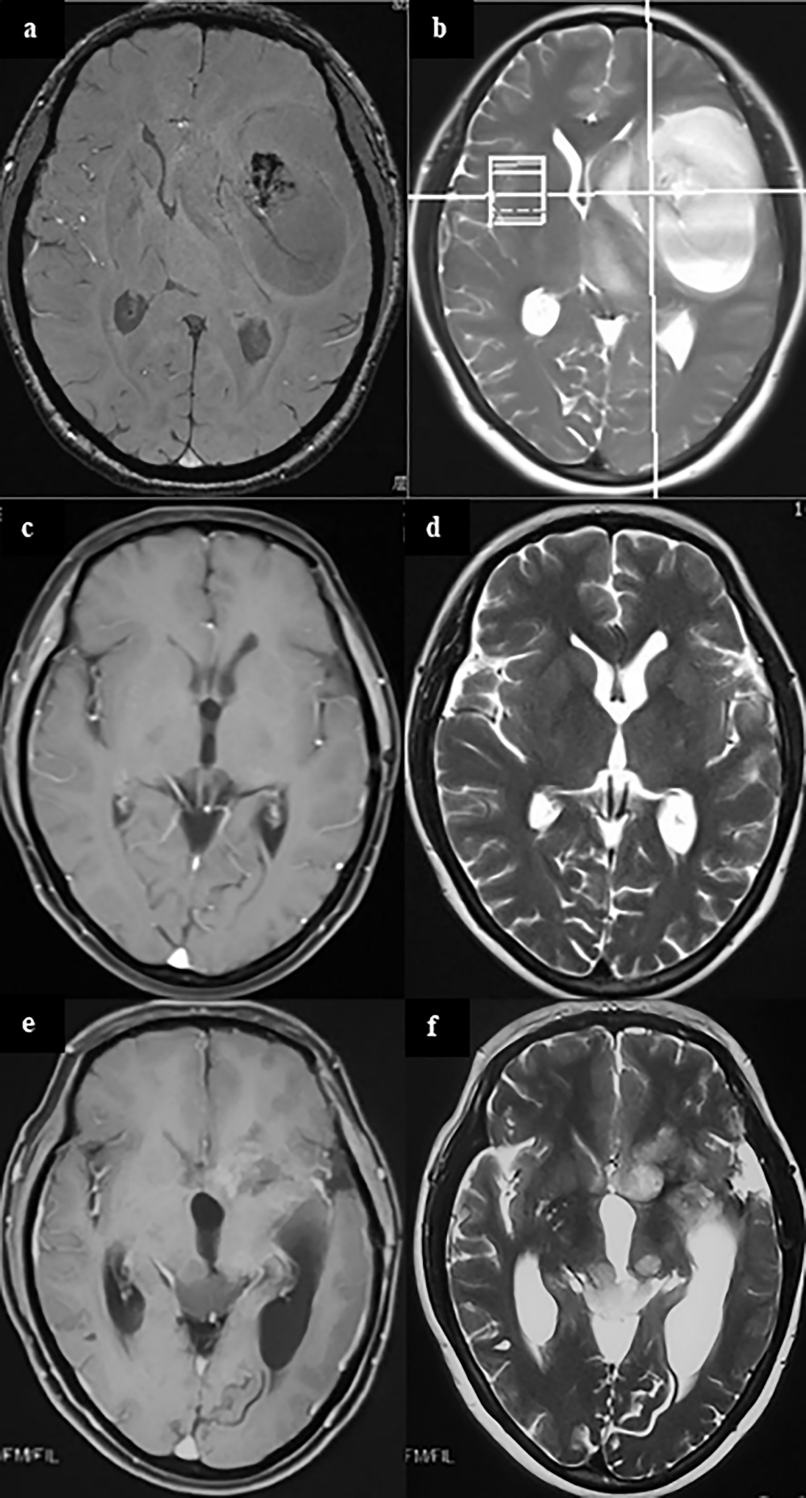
A case of metachronous multicentric glioma. The patient underwent MRI for headache and found a tumor in the left temporal insula (A, B). She was given partial resection of the tumor. Pathology showed GBM. Local radiation and temozolomide based chemotherapy were given after surgery. The tumor completely regressed in MRI 1 year after surgery (C, D). However, in follow-up 1.5 years after surgery, it was found that the patient had a recurrent lesion in the left caudate head and insula, and an ectopic lesion in the midbrain tectum (E, F). On sequential T2 and FLAIR MR images, there was no connection between the lesion in the midbrain tectum and in the right basal ganglia. In addition, cytologic test of CSF and MR of the brain and spinal cord did not find there was any evidence of CSF dissemination. Therefore, the lesion in the midbrain tectum is considered as a metachronous multicentric glioma in contrast with the initial tumor. The patient underwent ventricular-abdominal shunt surgery to relieve hydrocephalus and was given temozolomide chemotherapy again. She is still under follow-up (21 months after tumor resection). (a,c,e, MRI of T1 enhanced signal; b, d, f, MRI of T2 signal).
